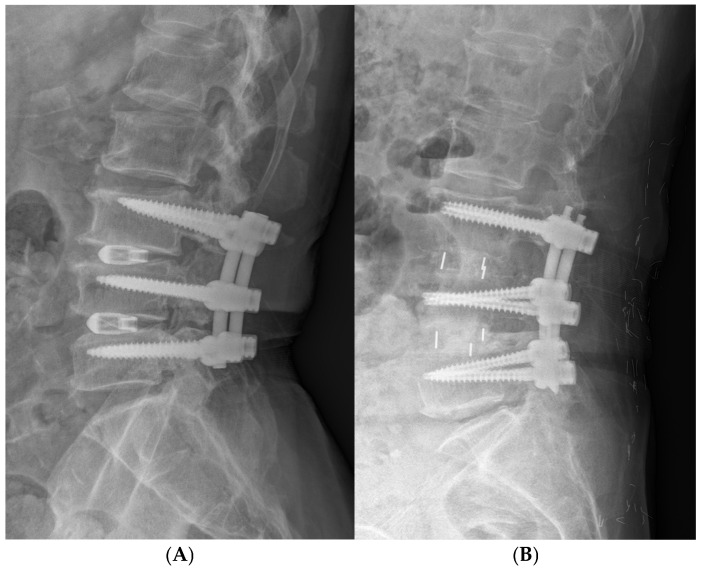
Standing lateral radiographs obtained at one-year postoperative follow-up after TLIF L3–4–5 (A) and LLIF L3–4–5 (B) and demonstrating maintained alignment and fusion status.
